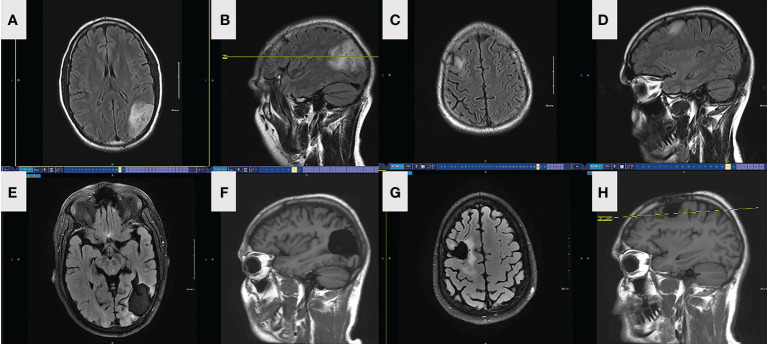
FLAIR axial (A) and sagittal (B) MRI depicting preop left parietal lesion in patient 1. FLAIR axial (C) and sagittal (D) MRI depicting preop right frontal lesion in patient 1. FLAIR axial (E) and sagittal (F) MRI depicting left parietal lesion and resection in patient 1, 18-month postoperatively. FLAIR axial (G) and sagittal (H) MRI depicting right frontal lesion and resection in patient 1, 18-month postoperatively.
